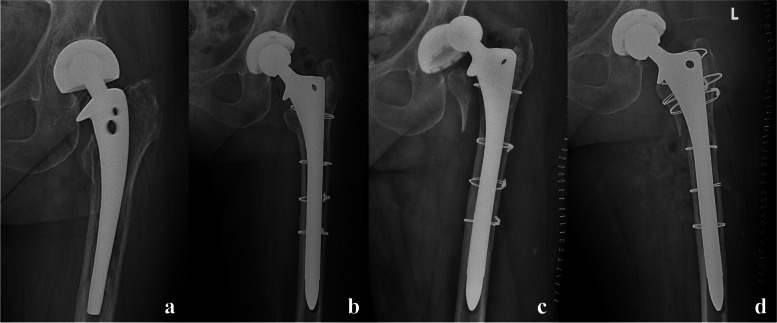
Another case of periprosthetic fracture. An 86-year-old female patient who developed severe osteolysis secondary to left hemiarthroplasty for femoral neck fracture 28 years ago and underwent total hip revision. PD was diagnosed 2 weeks after the operation, and a periprosthetic fracture occurred during the closed reduction process with the Allis maneuver. The prostheses were stable. Open reduction and internal fixation operations were performed under general anesthesia, and fracture fragments were fixed with wires. a. Preoperative X-ray film of the affected hip. b. AP view X-ray film of the affected hip immediately after revision. c. AP view X-ray film of the affected hip immediately after closed reduction with the Allis maneuver. d. AP view X-ray film of the affected hip immediately after open reduction and internal fixation
